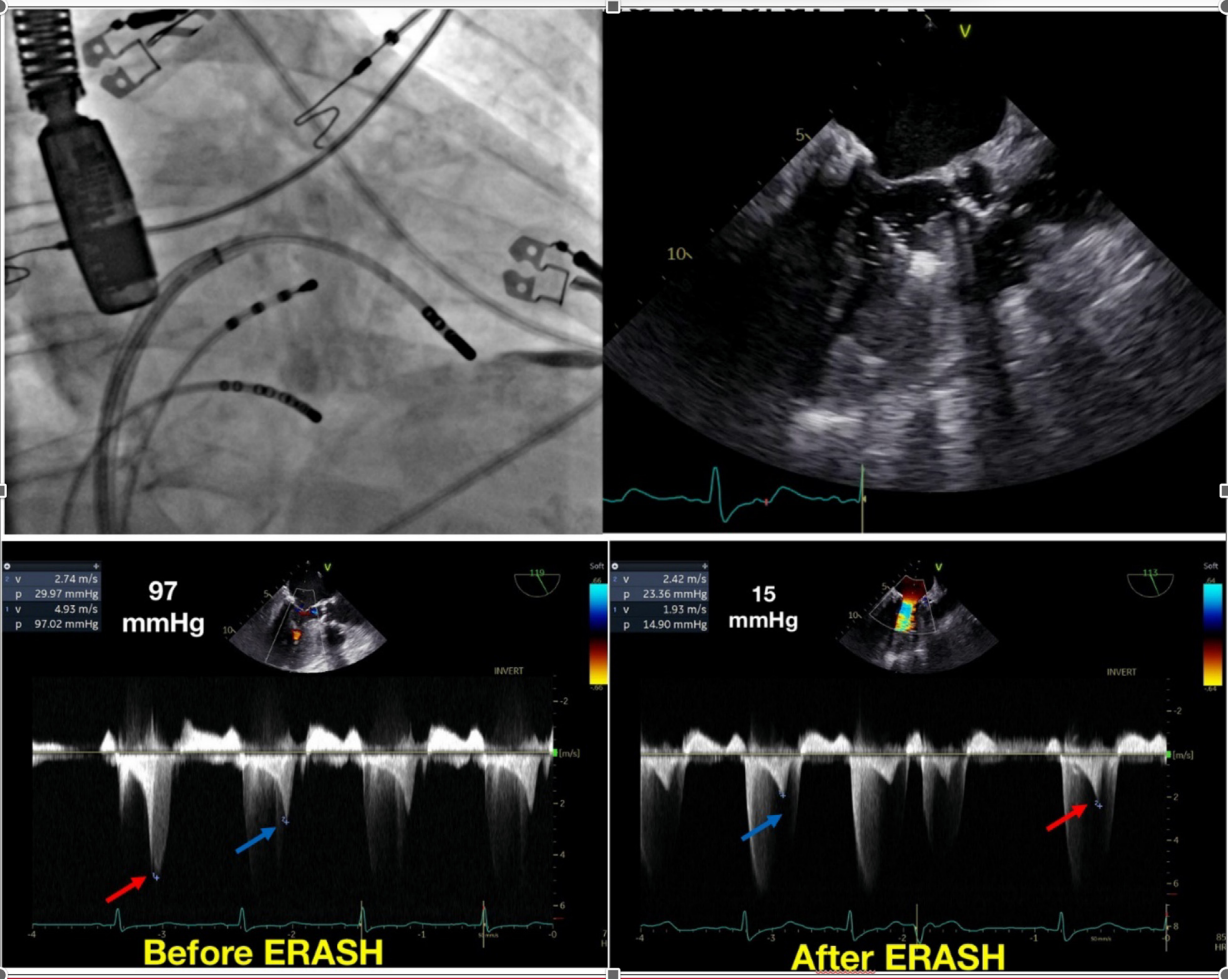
ERASH for Septal Preparation Before Transcatheter Aortic Valve Replacement (Top left) Fluoroscopic view of the ablation catheter. (Top right) Transesophageal echocardiogram view of catheter ablation of the left ventricular septum. (Bottom left) Maximum left ventricular outflow tract (LVOT) gradient after the ventricular premature beat (red arrow) and basal conditions (blue arrow) before ERASH. (Bottom right) Maximum LVOT gradient after the ventricular premature beat (red arrow) and basal conditions (blue arrow) after ERASH. ERASH = endocardial radiofrequency ablation of septal hypertrophy.
